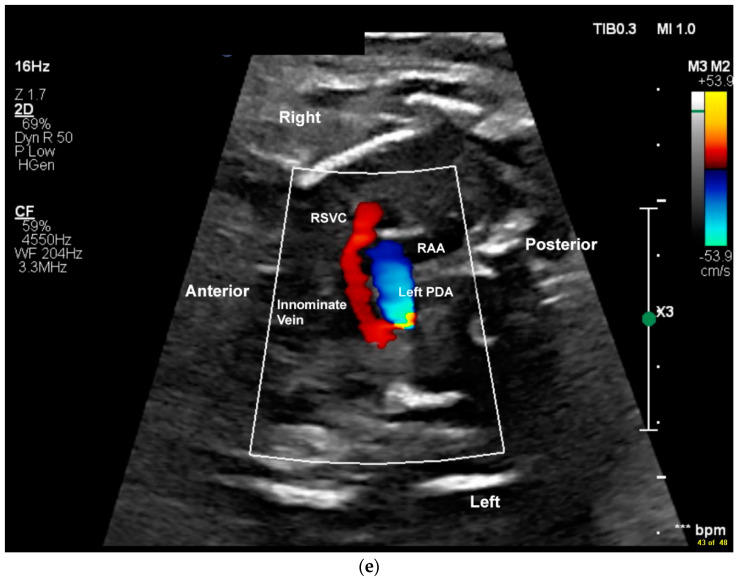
(a) Computed tomography angiography (CTA) coronal view showing the left ductus arteriosus dimple at the base of the left innominate artery. Right PDA, right sided patent ductus arteriosus; RPA, right pulmonary artery; MPA, main pulmonary artery; Left PDA, left-sided patent ductus arteriosus. (b) Anterior-posterior fluoroscopic view showing successful transcatheter ductal stenting with revascularization of the isolated left pulmonary artery (LPA). (c) Fetal echocardiogram color Doppler image at the level of right ventricular outflow tract (RVOT) showing the absence of bifurcation of the main pulmonary artery (MPA). LPA, left pulmonary artery; RPA, right pulmonary artery. (d) Abnormal 3-vessel and trachea (3VT) view with color Doppler. Viewed from right to left, these vessels include right superior vena cava (RSVC), aorta, main pulmonary artery (MPA) and left pulmonary artery (LPA). Right PDA, right-sided patent ductus arteriosus. (e) Fetal echocardiogram color Doppler image superior to the 3-vessel and trachea (3VT) view showing the left patent ductus arteriosus (PDA) origin and its course towards the left lung. This image also shows a right-sided aortic arch (RAA). RSVC, right superior vena cava.
